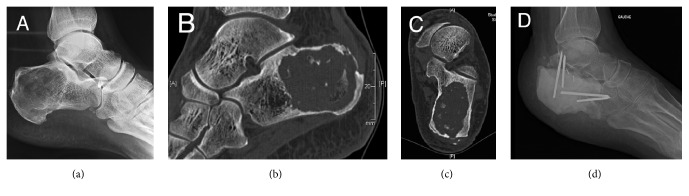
Clinical history: X-ray (a) and CT-scan (b and c) images of calcaneal chondrosarcoma showing a heterogeneous, lytic picture with intracystic calcifications. Visualization of a cortical rupture of the greater tuberosity with involvement of the Achilles tendon. Lateral X-ray (d) showing the spacer following calcanectomy with talocalcaneal and calcaneocuboid fixation.
